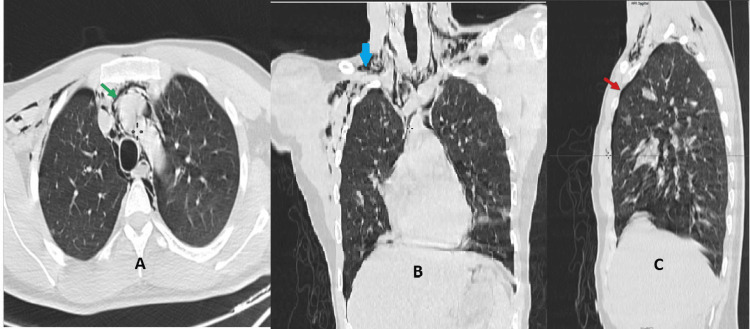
Thoracic CT imaging in axial (A), coronal (B), and sagittal (C) views showing pneumomediastinum (green arrow), subcutaneous emphysema (blue arrow), and minimal pneumothorax (red arrow).
